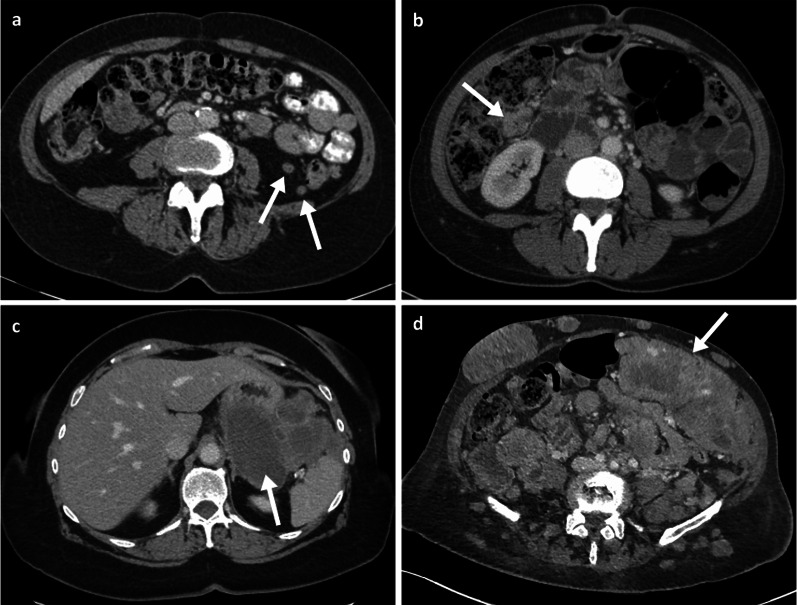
Peritoneal metastatic melanoma cases. a Oral and intravenous portal phase contrast-enhanced CT axial of 65-year-old left female with paracolic gutter nodules (arrows). b Portal phase contrast-enhanced CT axial of a 45-year-old female patient with a peritoneal deposit adjacent to the hepatic flexure (arrow). c Portal phase contrast-enhanced CT axial of a 73-year-old female patient with a large left upper quadrant necrotic peritoneal mass (arrow). d Portal phase contrast-enhanced CT axial of a 61-year-old female patient with a large left iliac fossa necrotic peritoneal mass (arrow) as well as numerous peritoneal, retroperitoneal and cutaneous nodules/masses
